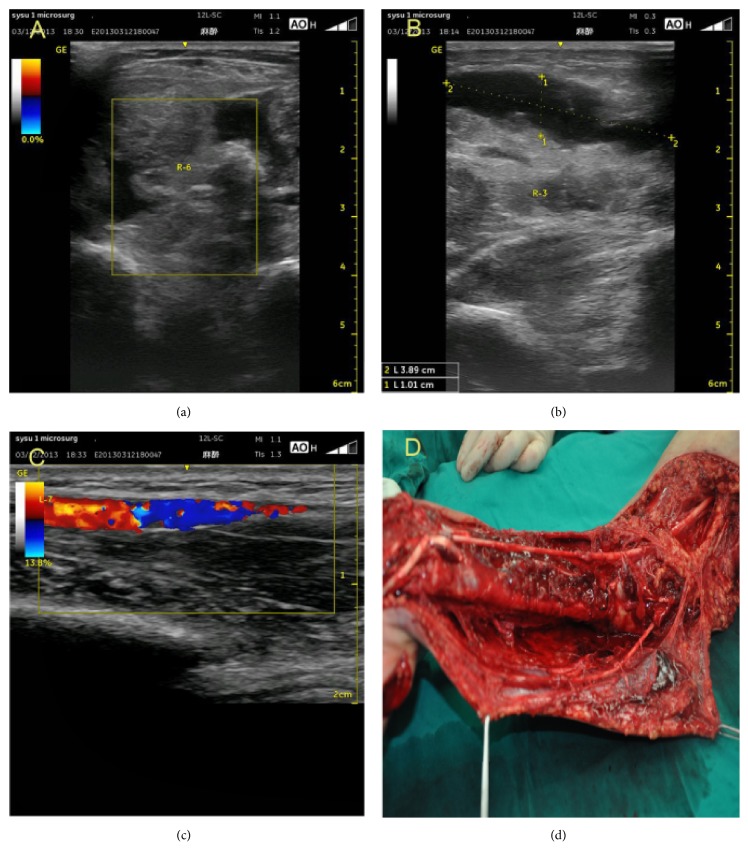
Ultrasound classification criteria for muscle injury—a case with a total score of 13 points. (a) Grade III lesions, complete muscle fibre rupture, ruptured muscle with retraction, fascial injury, the bell-clapper sign, torn muscle fragments floating in the serohematic fluid. (b) Hematoma presenting as an anechoic circumscribed lesion; the total muscle diameter is 39 mm. (c) Decreased arterial diameter and blood flow, partial arterial disruption. (d) The result of this patient after surgery: complete muscle fibre rupture, fascial injury, several hematomas present, partial but continuous arterial disruption.
